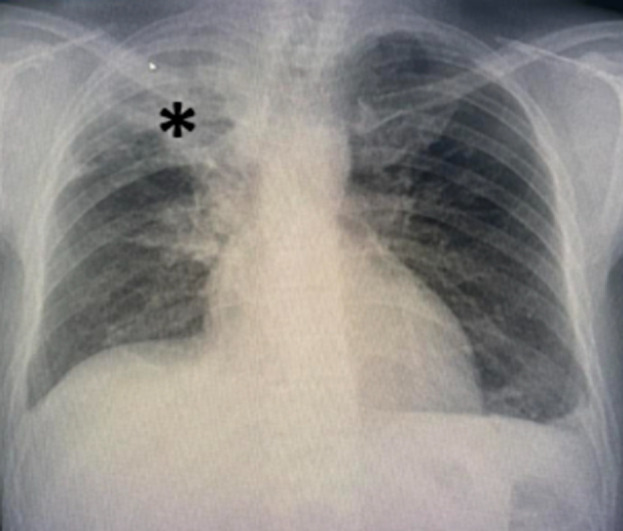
chest radiograph of the patient; right apical and supraclavicular heterogeneous opacities with interstitial and nodular infiltrates (*)
